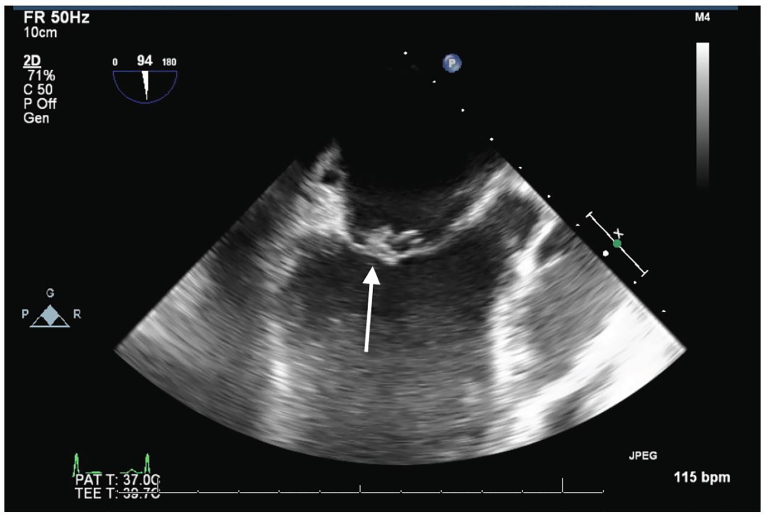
Transesophageal echocardiography Midesophageal longitudinal image of mitral valve showing vegetation (arrow) on anterior leaflet.
